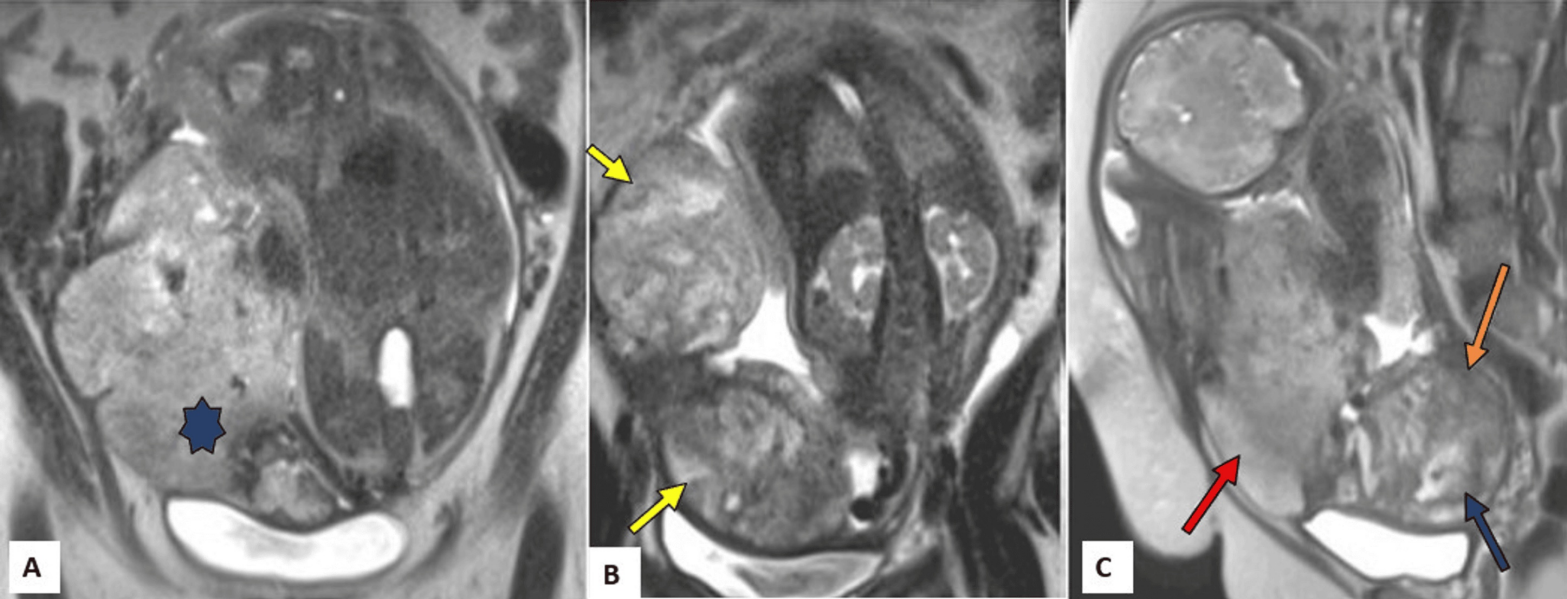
Magnetic resonance imaging of the pelvis with placenta: A and B, coronal sections; C, sagittal section. (A) Intrauterine foetal growth at 33 weeks with placentomegaly (blue star). (B) Focal uterine bulging areas with myometrium thinning along the lateral (upper yellow arrow) and anterior (lower yellow arrows) uterine walls. (C) Intraplacental haemorrhage (blue arrow) and retroplacental haemorrhage (orange arrow) along with uterine bulge in the anterior uterine wall (red arrow).
